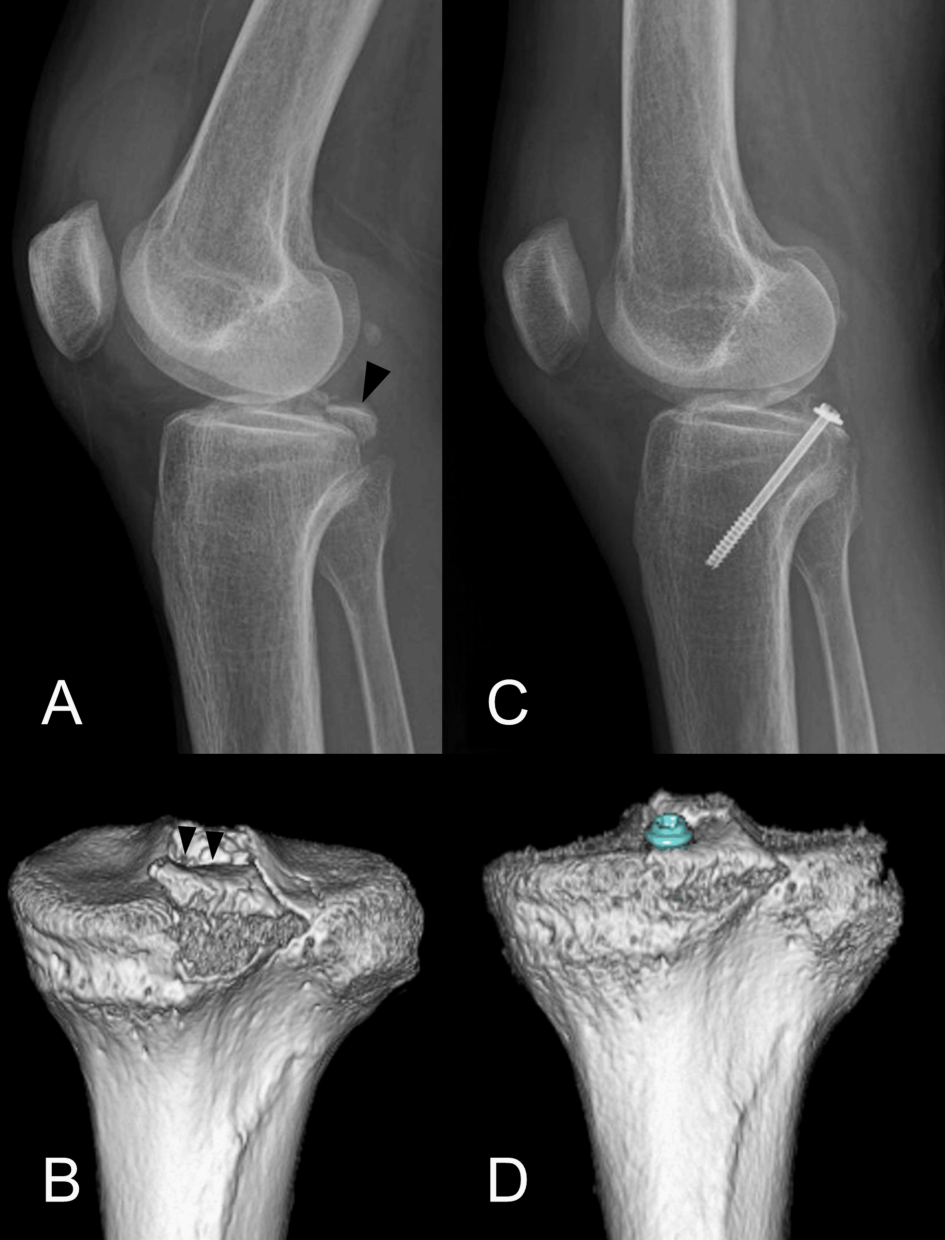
Case 3 (A) Preoperative lateral radiograph (arrowhead: bony fragment), (B) CT image (arrowhead: bony fragment). (C) Postoperative lateral radiograph and (D) CT image. CT, computed tomography; MRI, magnetic resonance imaging.
